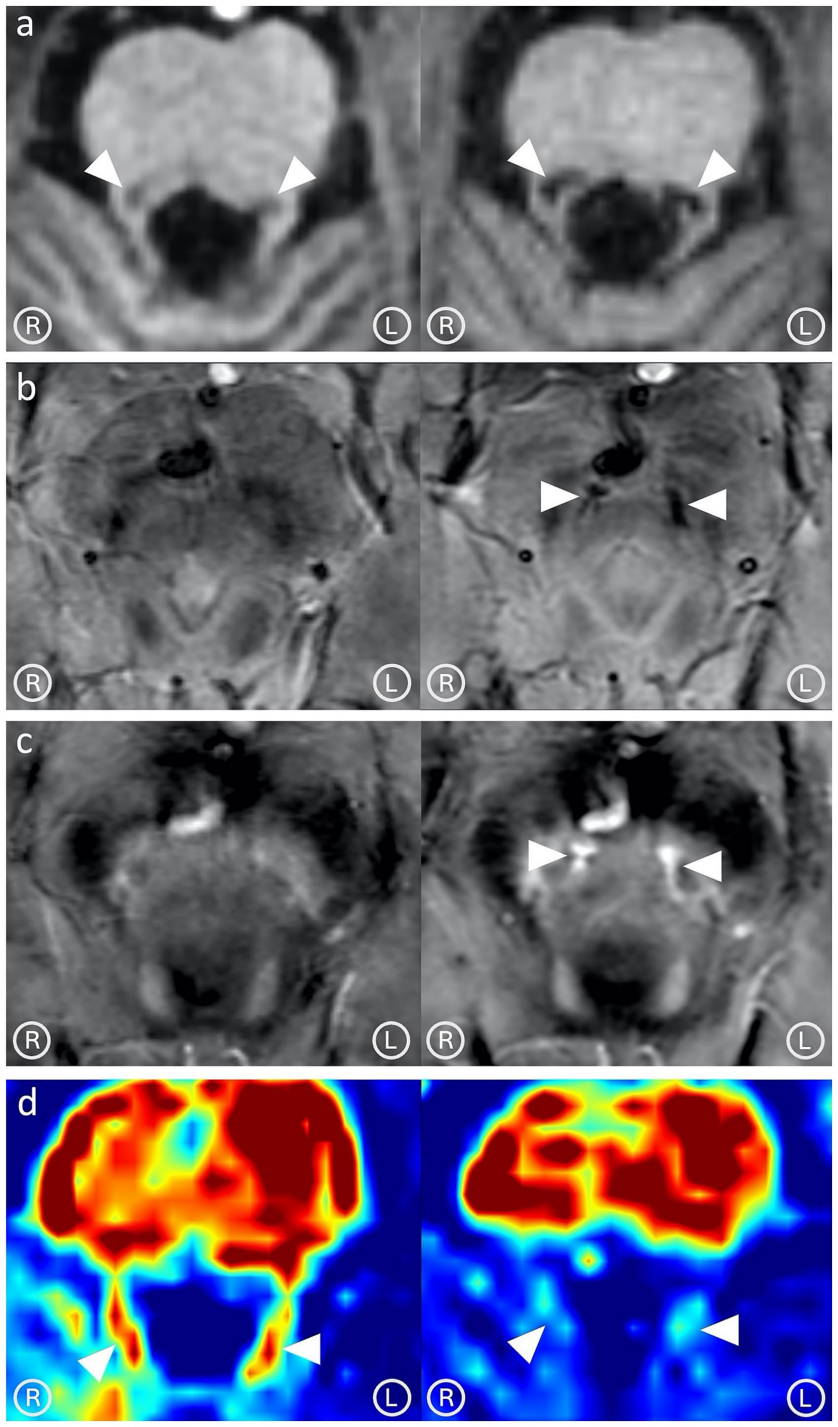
Comparison between the 7 T MRI (left column) and the follow-up MRI performed 8 months later (right column). Progression in the degeneration of the dentato-rubral tracts (DRTs) can be observed in T1-weighted images (a). Abnormally low signal in the T2*-weighted image (b) and increased susceptibility in the corresponding quantitative susceptibility map (c) appeared further cranially along the DRTs’ course, near the Wernekink commissure. A significant decrease in fractional anisotropy was observed in correspondence with the DRTs (d).
